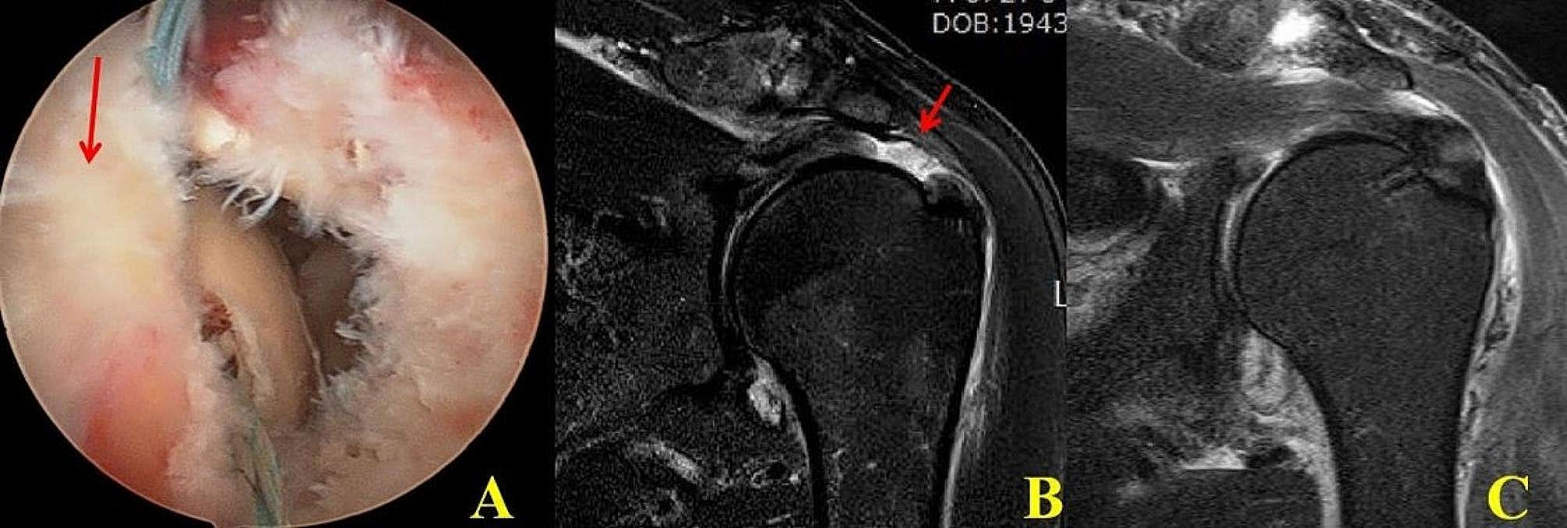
Classical transtendinous with remnant cuff tendon. (A) This classic type IIA configuration showed remnant rotator cuff was present on whole anteroposterior length of the greater tuberosity footprint. (B) On the preoperative and postoperative coronal MRI, transtendinous full thickness rotator cuff tear with 1 cm sized remnant cuff and tendon defect (arrow) was shown. (C) Postoperative follow-up MRI showed sufficient thickness well healed (good quality) repaired tendon
